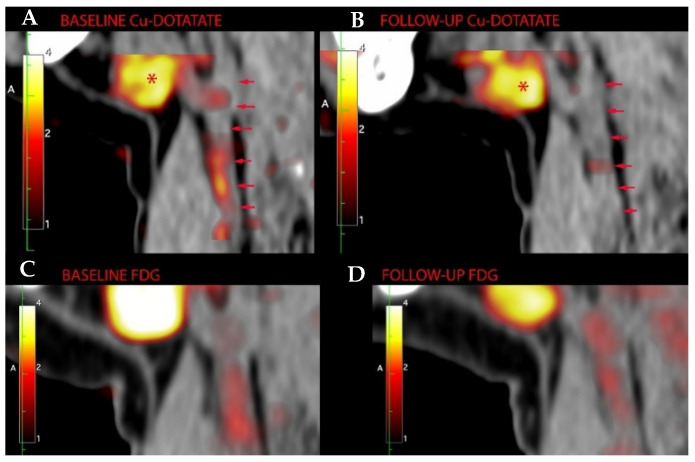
Sagittal [^64^Cu]DOTATATE-PET/CT images from a participant treated with liraglutide. The left carotid artery is outlined at (A) baseline (SUV_mean_ = 1.2) and (B) follow-up examination (SUV_mean_ = 0.92). * Physiological uptake in submandibular gland. The lower panels show [^18^F]FDG uptake from the same participant for comparison at (C) baseline and (D) follow-up.
